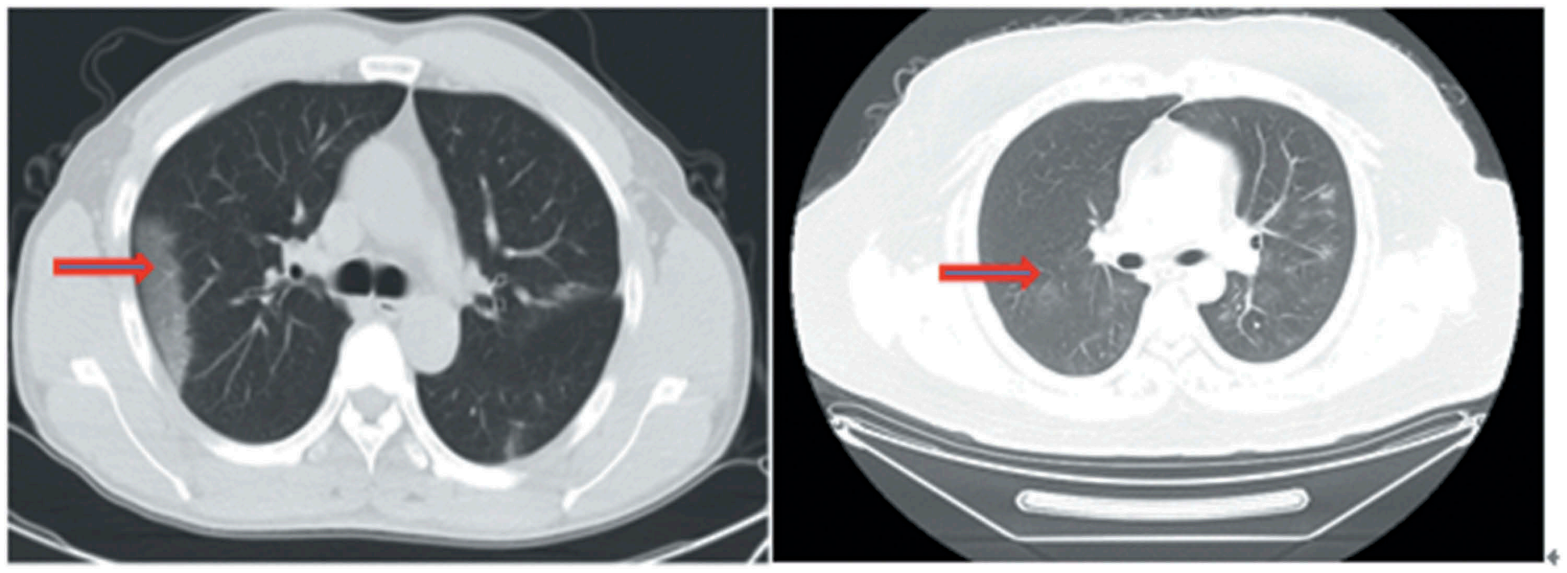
Typical changes in non-contrast enhanced chest CT scan. Multiple peripheral patchy ground-glass opacities with obscure boundary were seen in bilateral multiple lobes. Condensation shadow was observed on the lower right lobe.
